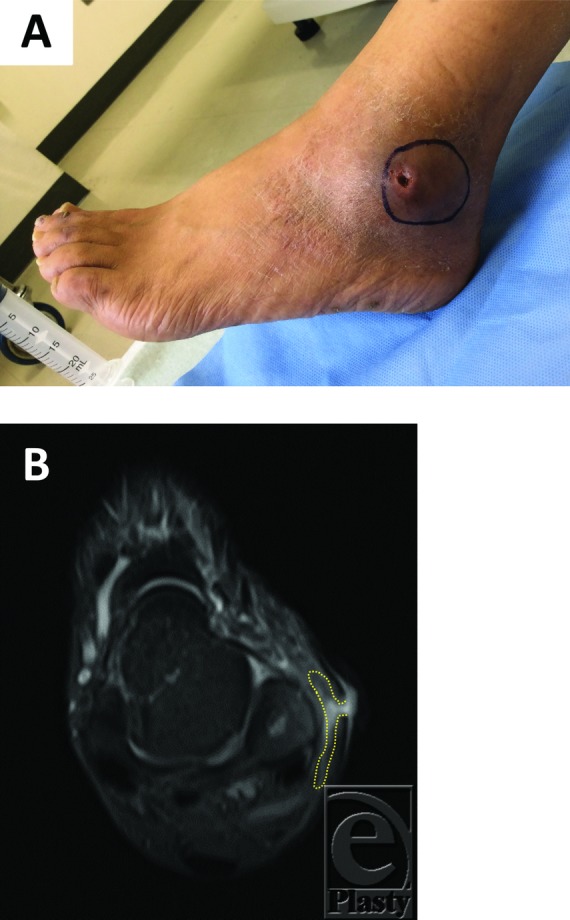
(a) Macroscopic finding showed that a fistula with subcutaneous cavity measuring 4 × 3 cm was observed in the left lateral malleolus. The mark indicates the margin of the subcutaneous cavity. (b) Computed tomographic findings of the left lateral malleolus. The yellow dotted line indicates the margin of the subcutaneous cavity.
